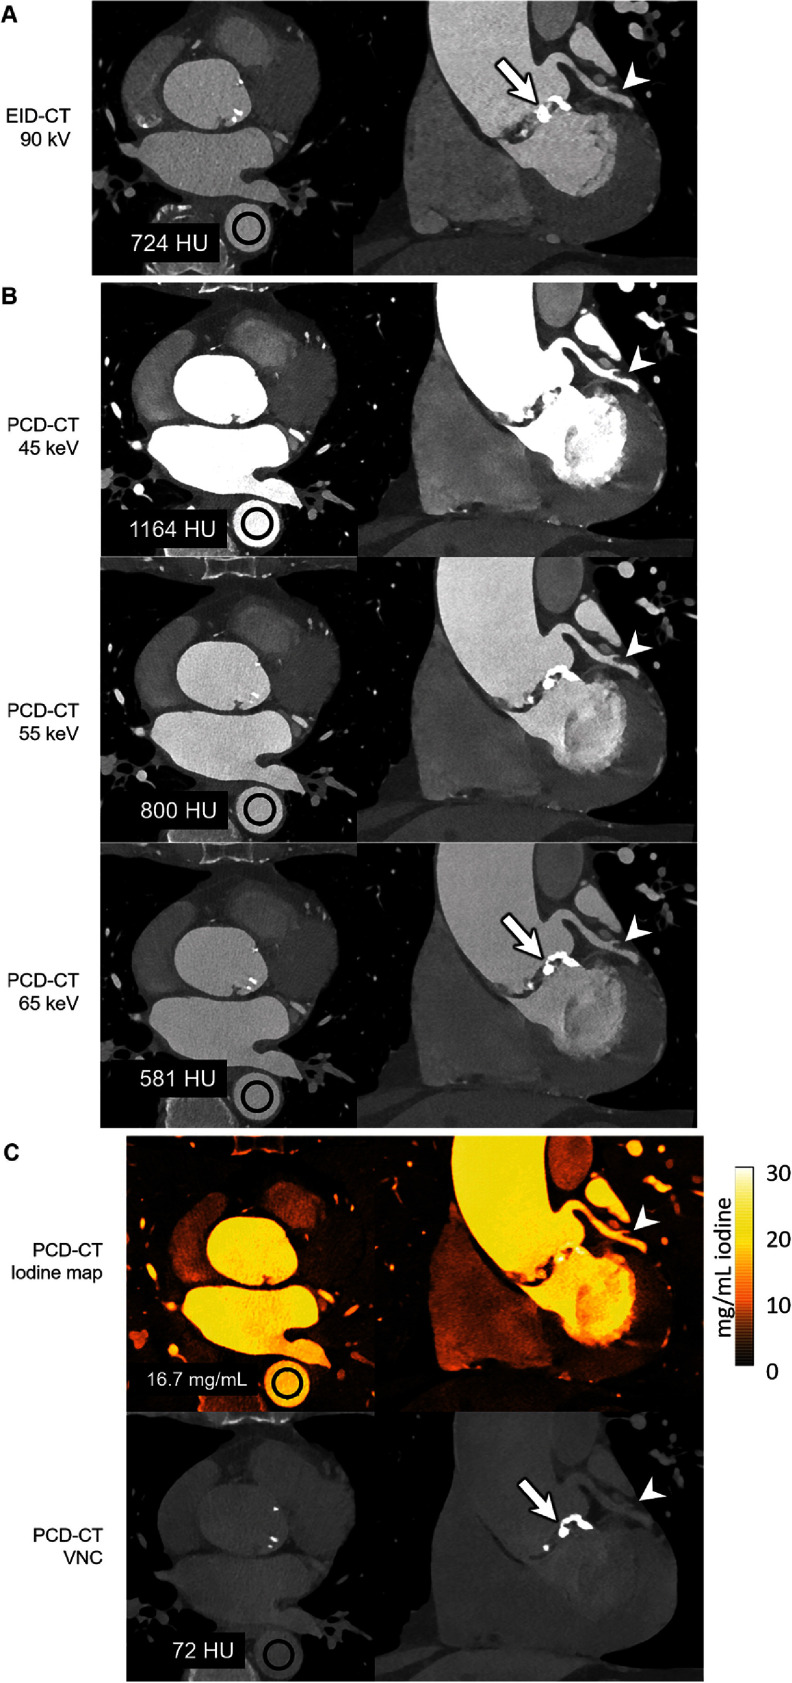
Images in a 71-year-old man scanned with (A) energy-integrating detector (EID) based CT and (B), (C) PCCT. The multienergy capabilities of the PCCT system allowed the creation of low-energy virtual monoenergetic images (VMIs) (B), showing increased iodine signal and improved delineation of coronary arteries compared to single-energy EICT (A). The bottom row (C) also includes a Virtual Non-Contrast (VNC) image, created by subtracting iodine from the contrast-enhanced dataset, which effectively mimics a non-contrast image. Reconstruction kernels and display settings varied between EICT and PCCT. Reproduced with permission from Rajendran *et al* (2022).
